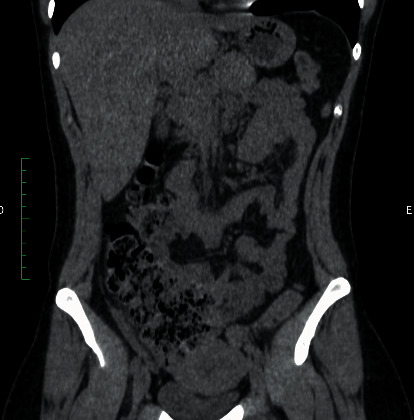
Computed tomography—coronal section.
